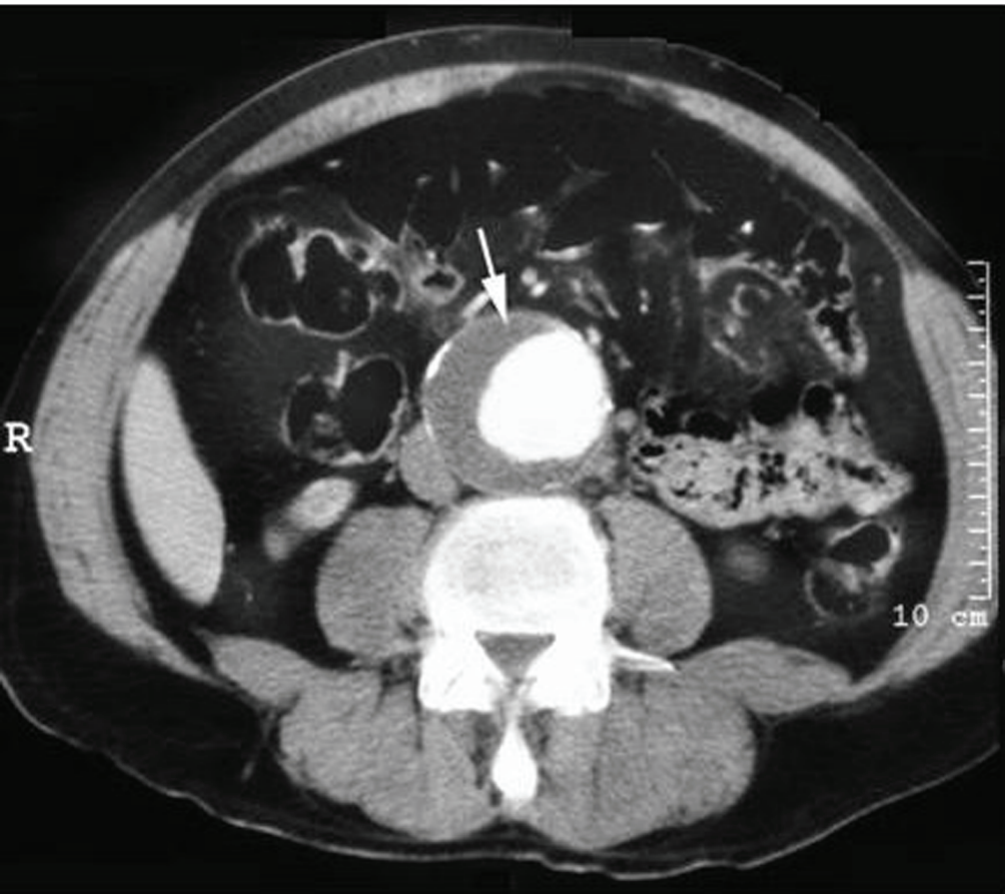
CT scan confirming 6.6 × 5.8 cm infrarenal aneurysm with thrombus in lumen.
